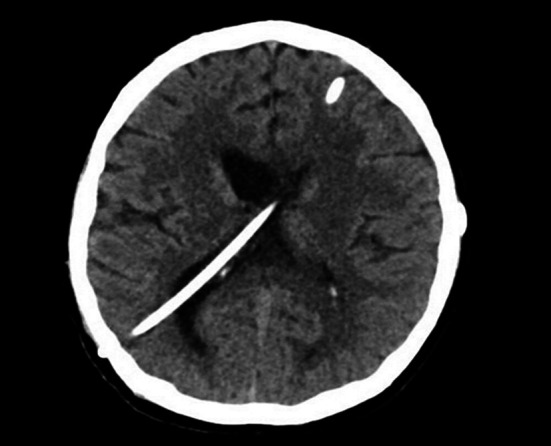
Plain CT brain scan axial image demonstrates bilateral functioning VPS.
